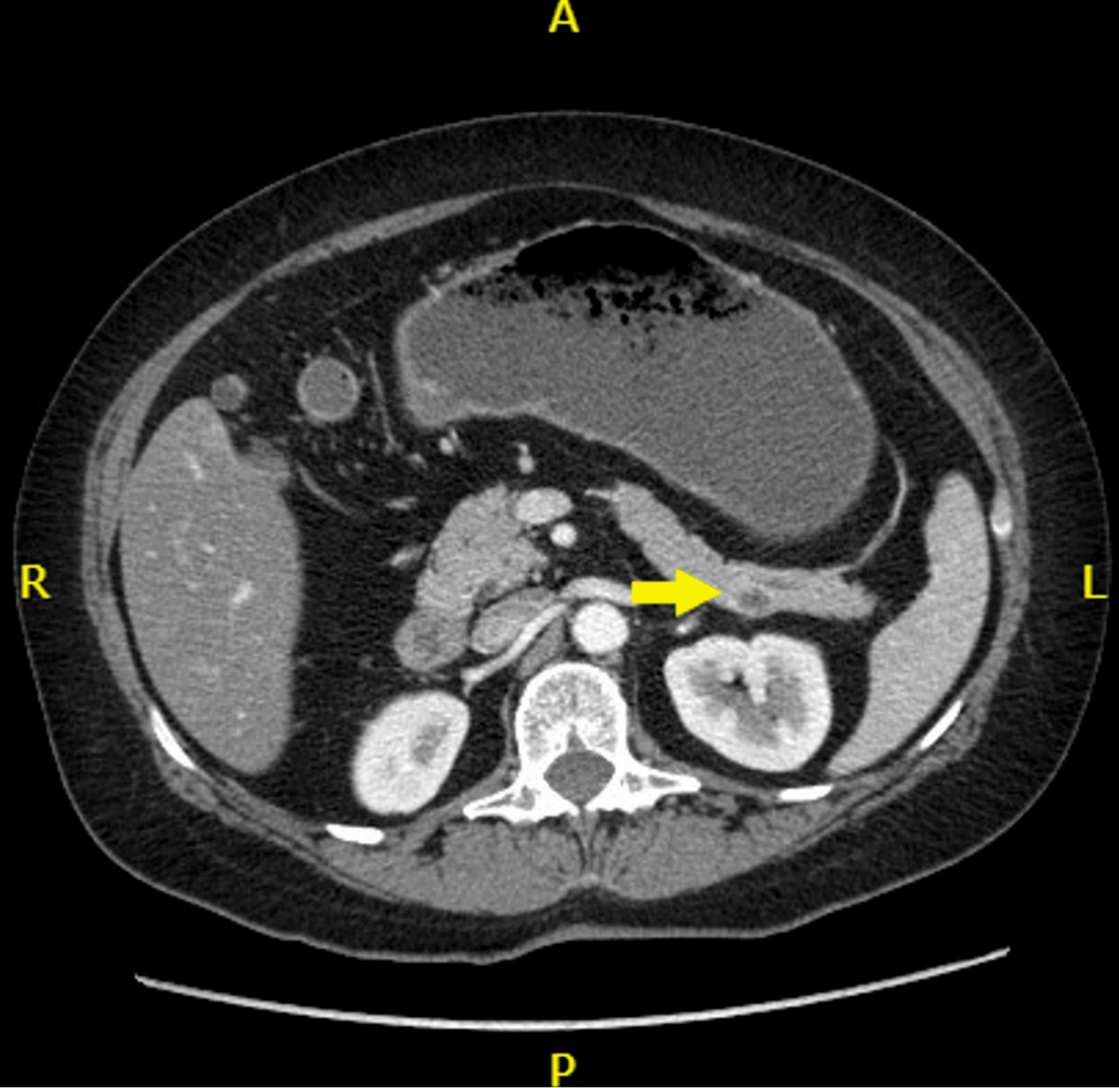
Abdominal CT performed in 2022 CT scan showing 9 x 5 x 5mm solid mass in the tail of the pancreas (arrow). Given the solid, cystic, well-encapsulated nature of the mass, clinical suspicion for malignancy was low. Additionally, the lack of involvement with nearby structures to include the pancreatic duct, and the patient's asymptomatic presentation were accounted for in the decision-making process to reevaluate the mass with close follow-up CT: computed tomography
